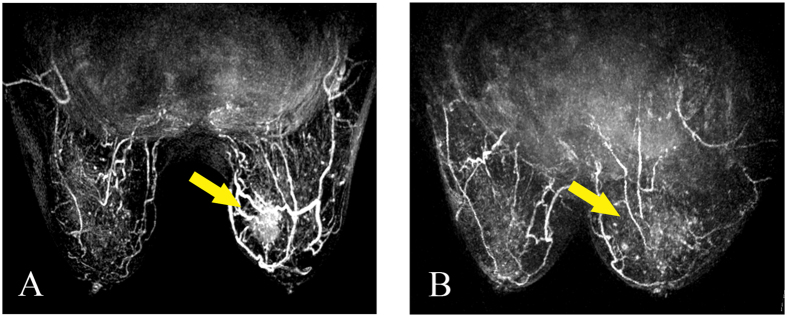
(A,B) Peripheral vessel changes on DCE-MRI before and after completion of NAC for breast cancer (female, 64 years old, invasive ductal carcinoma, grade II). (A) Before NAC, DCE-MRI image showed apparently richer and coarser peripheral vessels of the tumor. (B) After the completion of NAC, the peripheral vessels and the tumor seemed to disappear compared to the contralateral breast.
